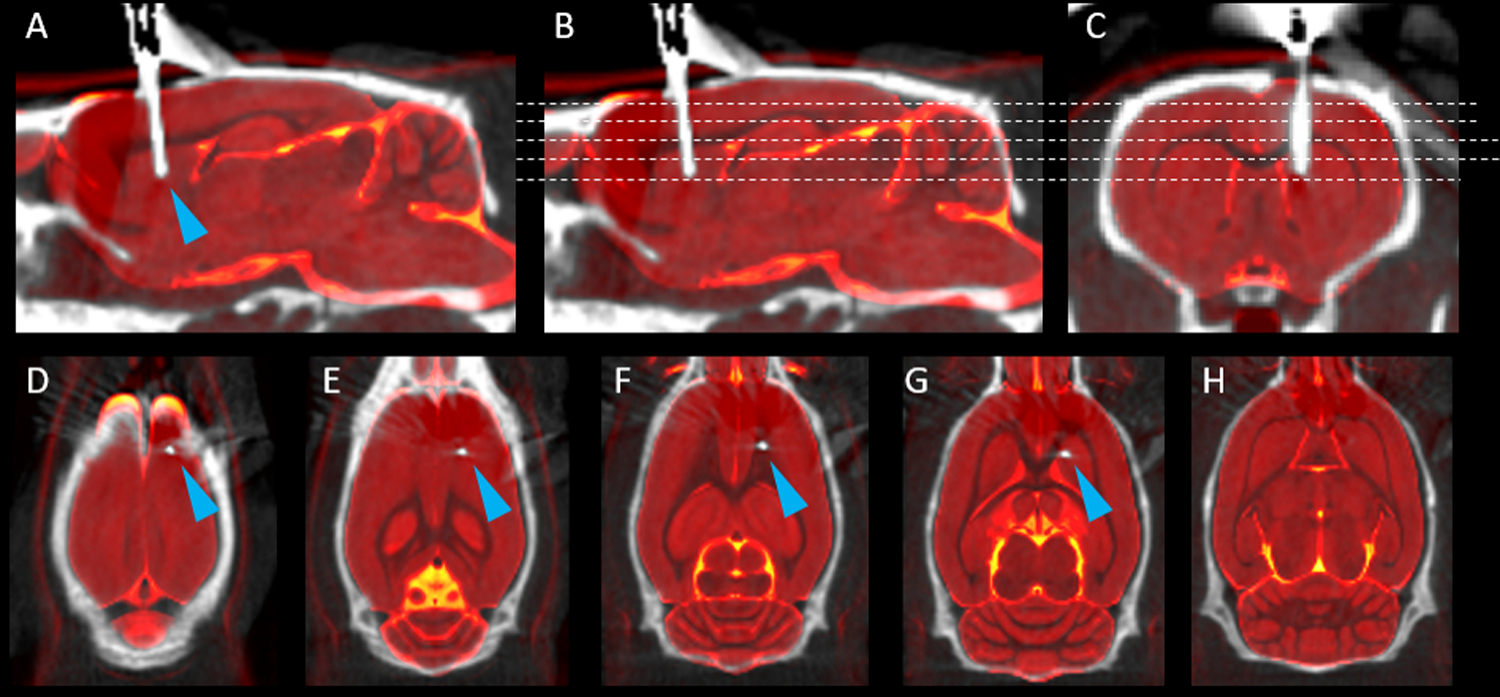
(A-C) Sagittal and coronal CT slices (greyscale) overlayed with a standard high resolution structural MR (redscale) showing the location of the neural interface (blue arrow) in the right DS. (D-H) Horizontal slices from the planes shown as dashed lines in (B and C), showing the trace of the neural interface through the brain.
